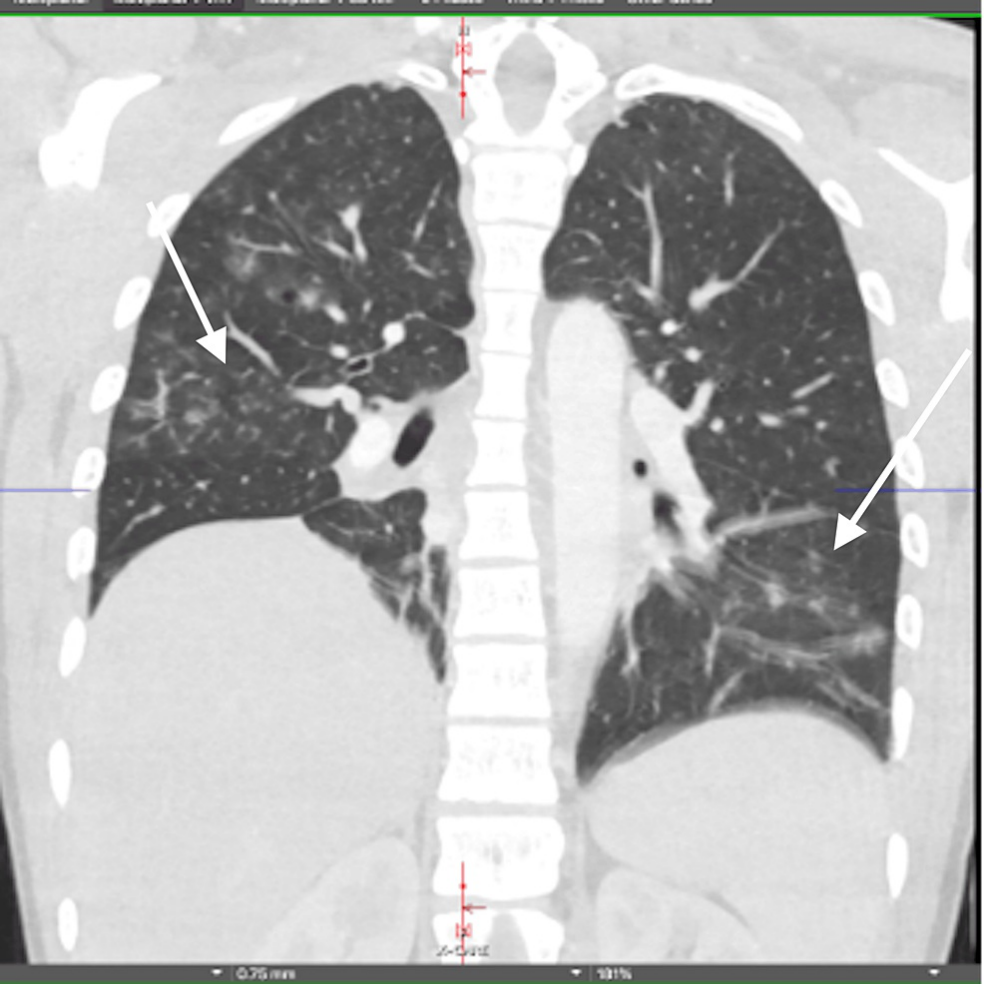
CT of the chest revealed bibasilar atelectasis and multifocal ground glass opacities within bilateral lungs, consistent with COVID-19 pneumonia.
